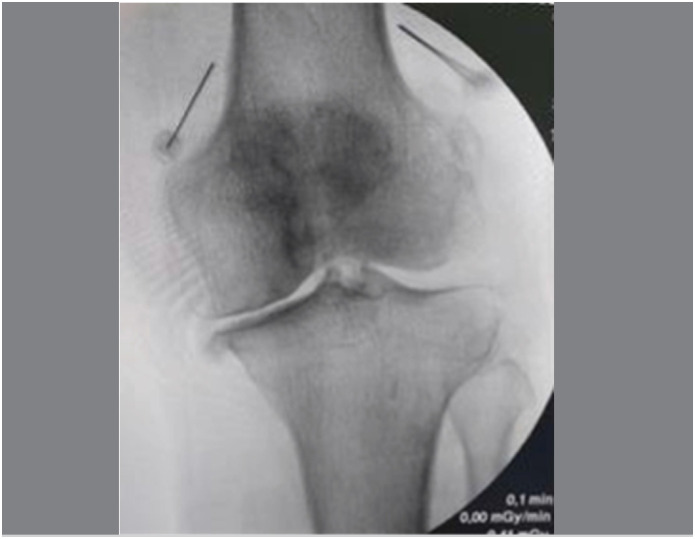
Positioning in AP radiography for thermoregulation of the superior medial genicular nerve and superior lateral genicular nerve. AP x-ray incidence of medial and lateral superior genicular nerves radiofrequency.
